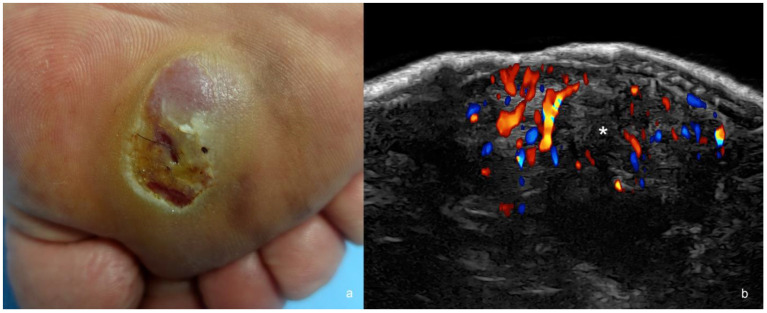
(a,b). Melanoma. (a). Clinical image in the plantar region. (b). Color Doppler ultrasound image at 24 MHz (transverse view) demonstrates hypoechoic dermal and hypodermal mass (*) with irregular borders and prominent hypervascularity.
